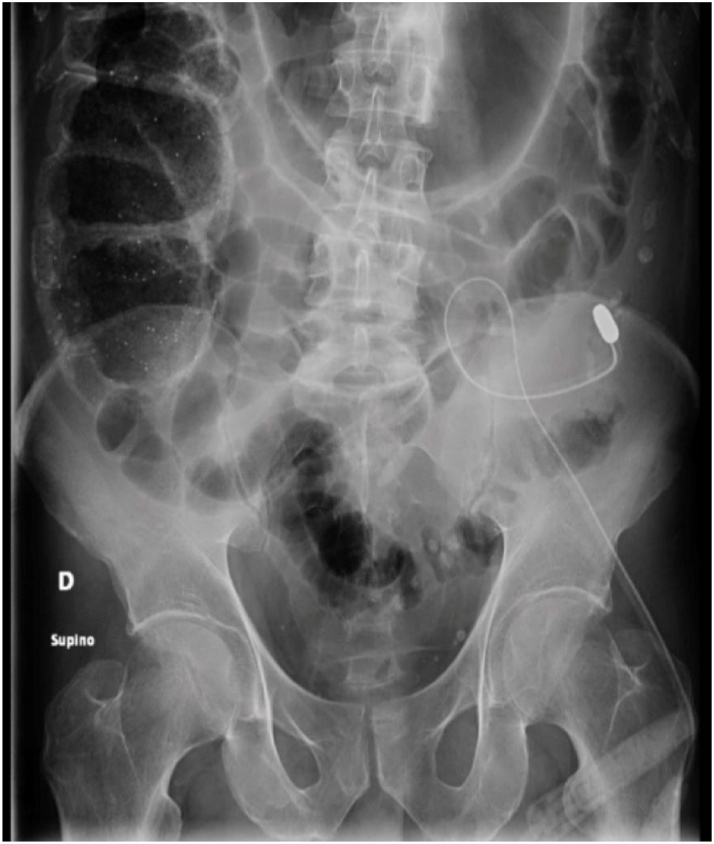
X-ray showing the tungsten tip of the catheter in the left iliac fossa, with intestinal distension.
